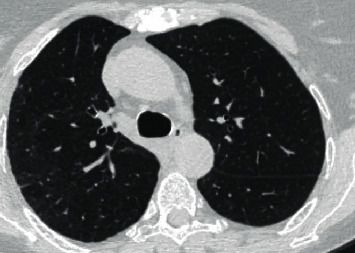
Repeat chest CT at six months shows continued improvement in right lobe infiltrate.
